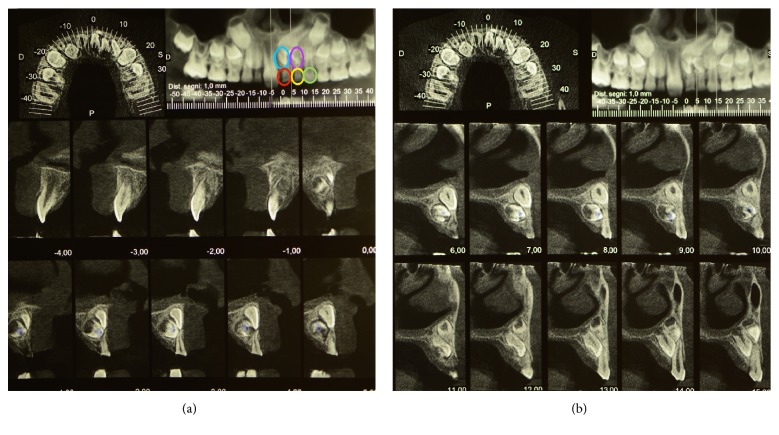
(a) CBCT of the patient showing 3 deciduous teeth (61 in red circle, 62 in yellow circle, and 63 in green circle) and 2 supernumerary teeth (blue and violet circles). (b) CBCT of the patient showing displacement of permanent teeth 21 and 22.
